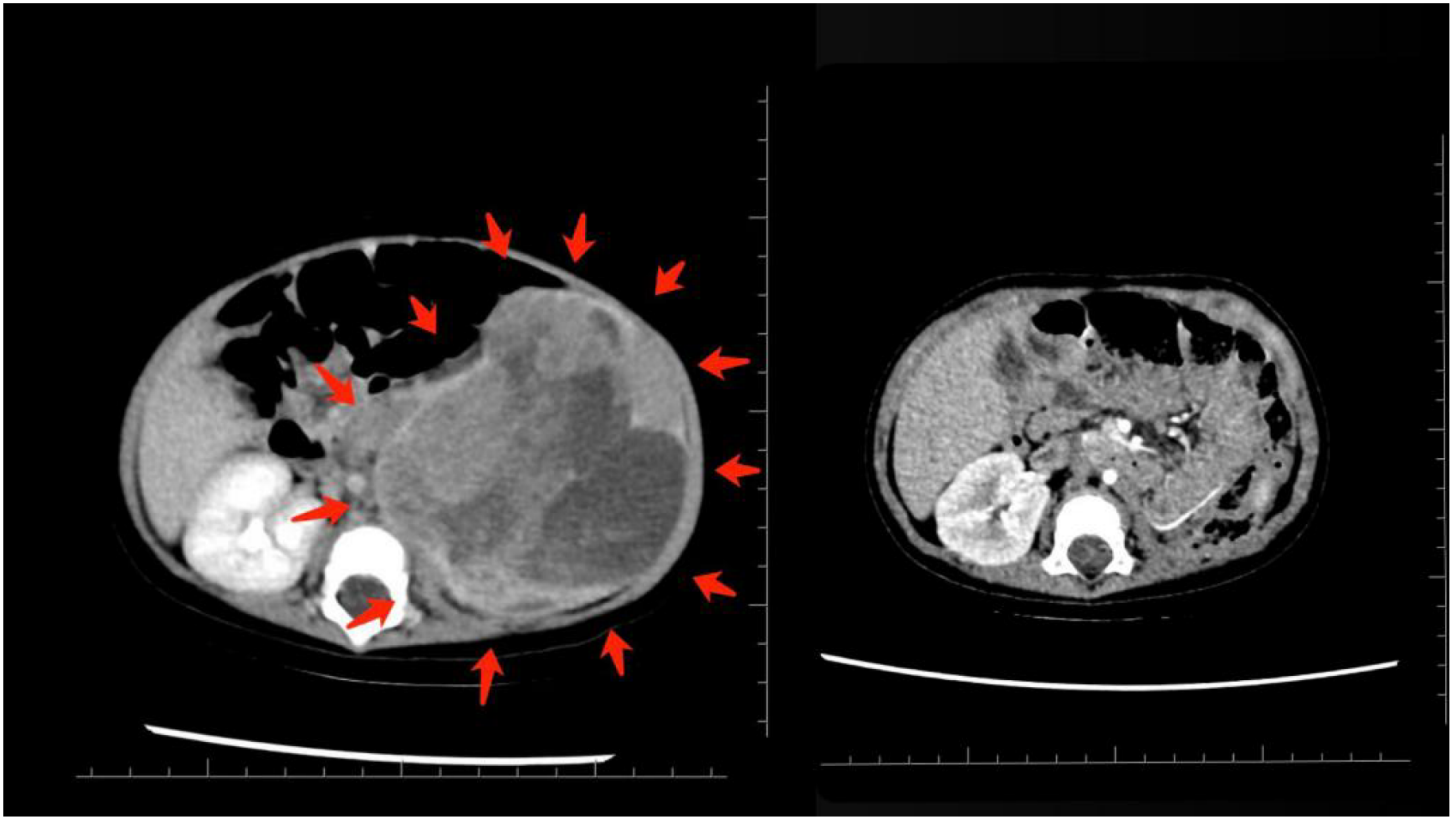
Contrast-enhanced CT scans of the abdomen. Right panel: preoperative imaging demonstrating large heterogeneous masses involving the left kidney and adrenal region. Left panel: postoperative scan after left nephrectomy showing no contrast-enhancing residual mass in the surgical bed.
